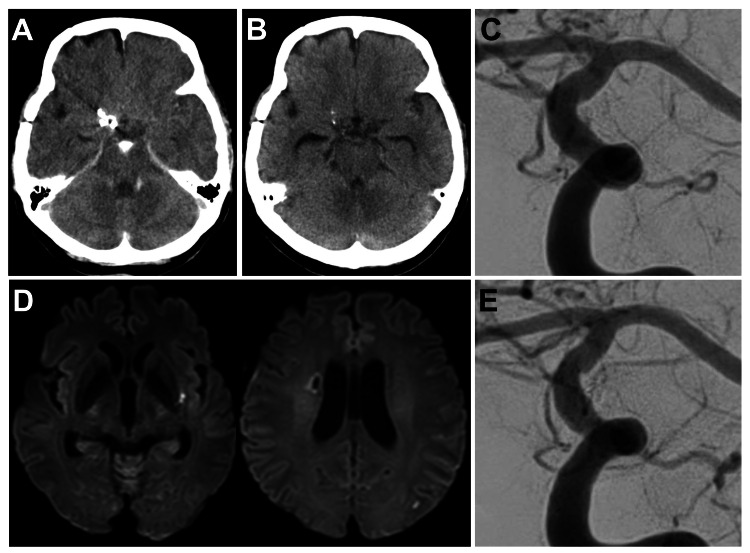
Imaging findings after endovascular surgery. (A) CT immediately after surgery shows a high-density area in the interpeduncular cistern. (B) CT the day after surgery revealed that the high-density area in the subarachnoid space has almost disappeared. (C) Left internal carotid angiography of working projection the day after surgery shows no extravasation of contrast media and a slight neck remnant of the aneurysm. (D) Diffusion-weighted MRI of the head the day after surgery shows a small high intensity spot outside the left putamen and in the left occipital lobe. (E) Left internal carotid angiography of working projection six months after surgery showing complete occlusion of the aneurysm.
